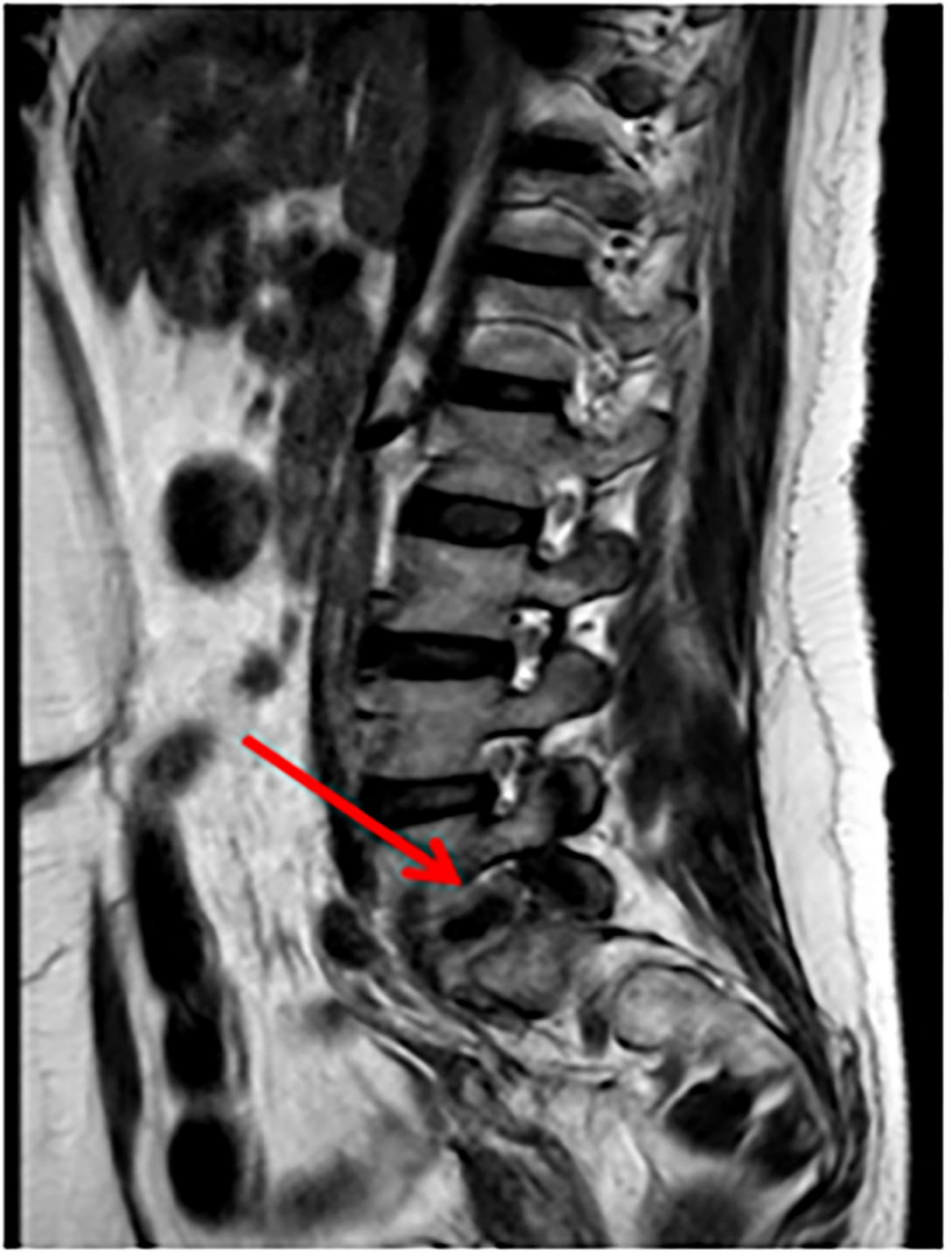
Pelvic MRI: enhancement of soft tissues surrounding the L5-S1 vertebrae (arrow). MRI, magnetic resonance imaging.
